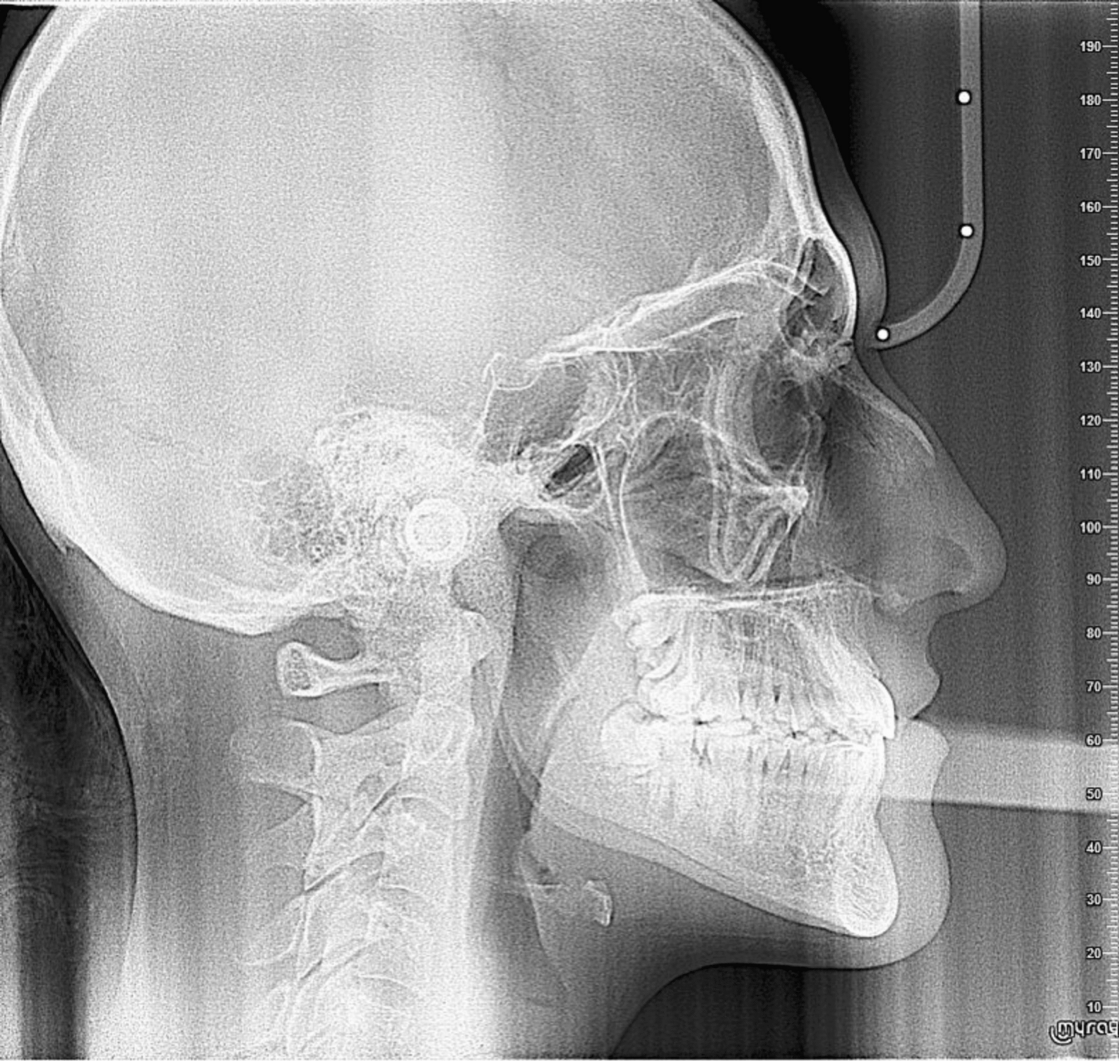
Lateral cephalometric radiograph taken after treatment. Lateral cephalometric radiograph demonstrating the final skeletal and dental relationships.
